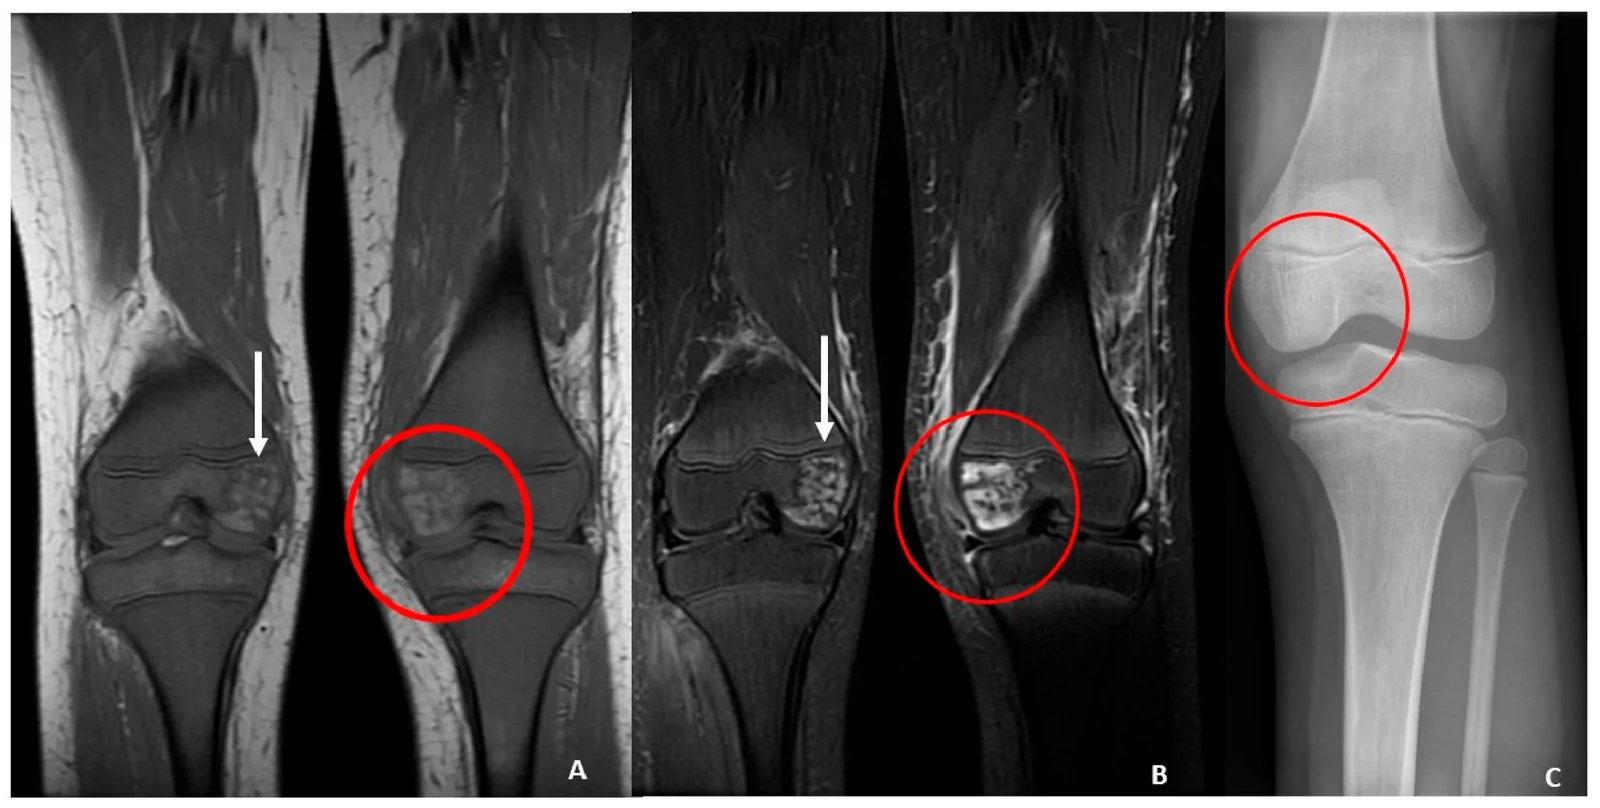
Magnetic resonance imaging (MRI) and radiographic findings of osteonecrosis in a child with sickle cell disease and left knee pain. Red circles in panels (A,B) show T1- and T2-weighted MRI images demonstrating an osteonecrotic lesion involving the left femoral medial condyle. In addition, there is a developing osteonecrotic lesion in the right femoral medial condyle (white arrow). Panel (C) shows the corresponding plain radiograph in the same patient, which demonstrates no visible abnormality in the left femoral medial condyle (red circle), highlighting the superior sensitivity of MRI for detecting early osteonecrosis.
